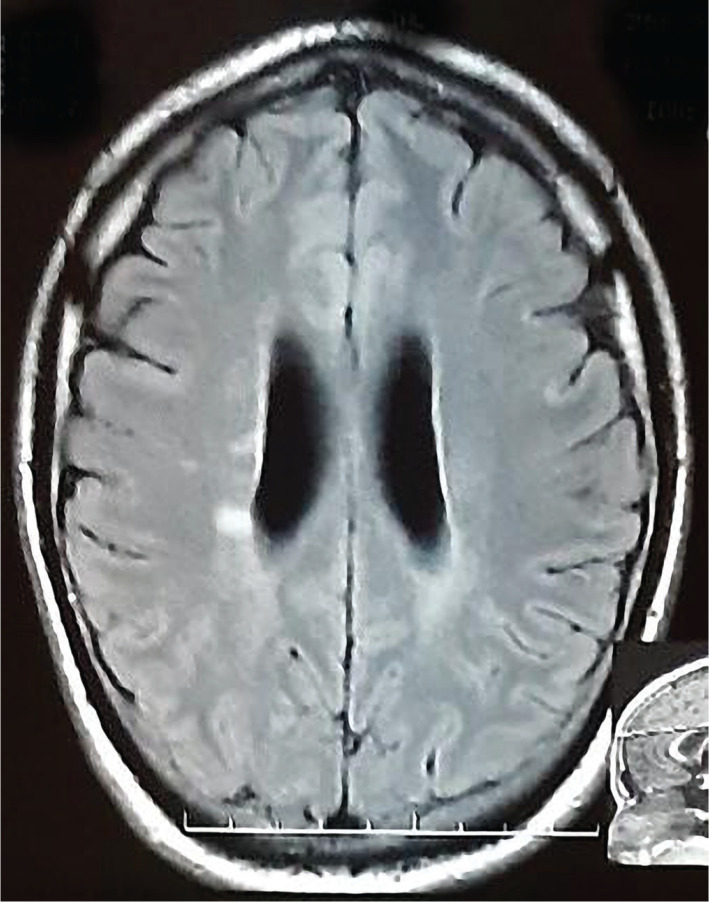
Axial fluid-attenuated inversion recovery (FLAIR) MRI section revealing an acute hyperintense contrast-enhancing lesion in the right periventricular region.
